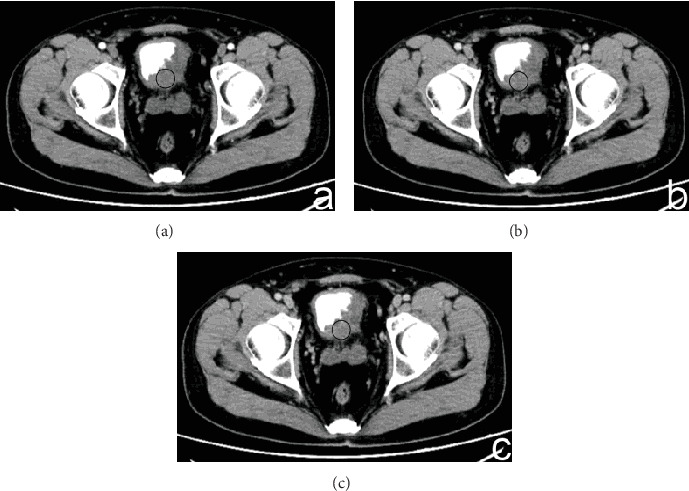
(a–c) A 56-year-old man had bladder cancer that presented as mound-like lesions in the left lateral wall of the bladder, including the trigone. The enhanced CT values of the lesions in the arterial, venous, and delayed phases were 77.2, 83.4, and 79.7 HU, respectively. The enhanced CT values of the bladder wall in these three phases were 31.4, 39.2, and 34.5 HU. The relative enhanced CT values (calculated as enhancement CT values of the lesion − enhancement CT values of normal bladder) in the arterial, venous, and delayed phases were 45.8, 44.2, and 45.2 HU, respectively.
